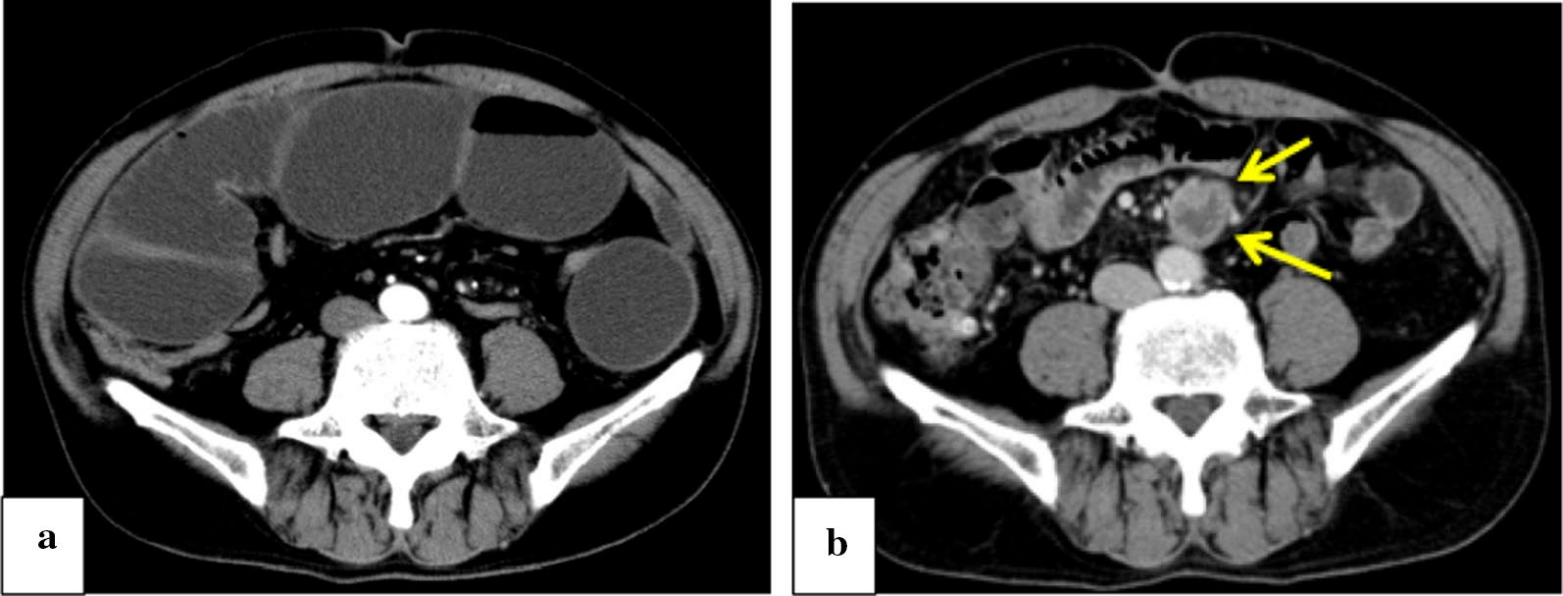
a Metastasis in the para-aortic lymph node was not observed by the preoperative CT scan. b The para-aortic lymph node metastasis was found by a follow-up abdominal contrasting CT scan one and a half years after the operation. The arrow shows the lymph node metastasis
